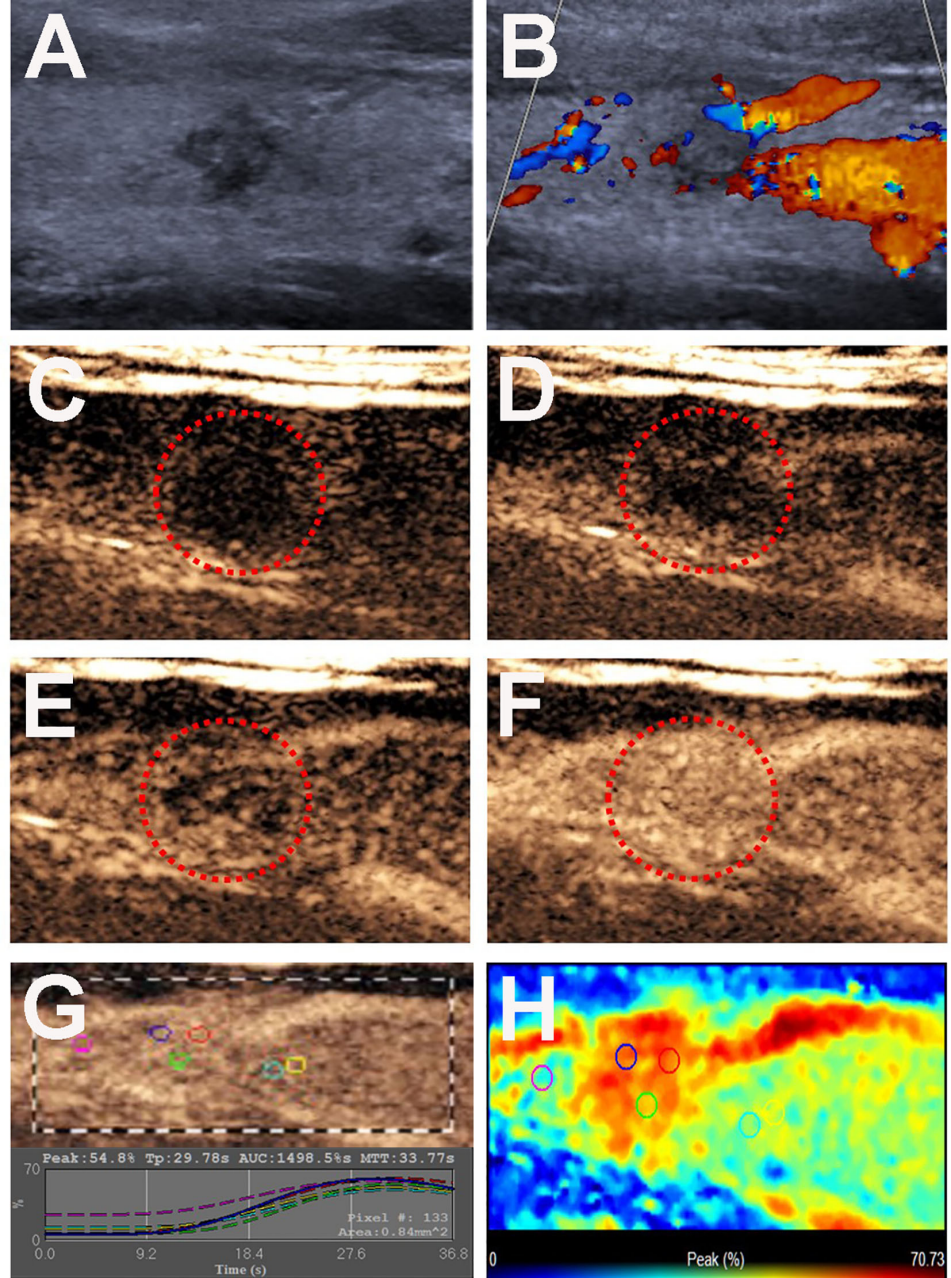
Conventional US and CEUS images of a 26-y-old male PTC patient with Hashimoto’s thyroiditis of the right thyroid lobe without CLNM. (A) B-mode US image revealing a 8.0-mm solid thyroid nodule with a taller than wider shape, hypo-echogenicity, ill-defined margins. (B) Doppler image revealing abundant peripheral vascularity and no obvious internal vascularity. (C–F) CEUS images revealing a centripetal homogeneous hyperenhancement of thyroid nodule from the periphery to the center, at (C) 2s, (D) 20s (wash-in), (E) 22s and (F) 34s (time to peak). (G) TICs of the thyroid nodule and peripheral thyroid parenchyma with different ROIs (different color circles). (H) Parametric color map indicating the PI values for the nodule was higher than that of adjacent thyroid parenchyma.
